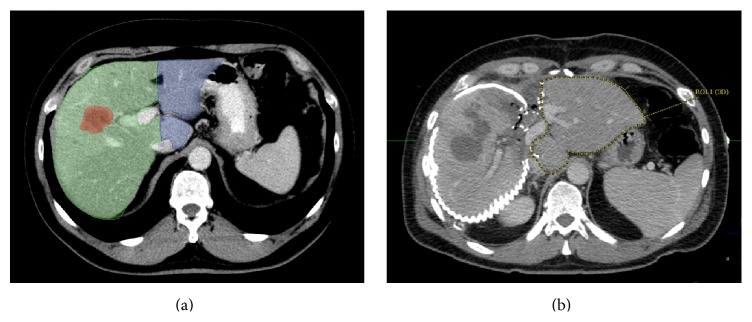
Pre- and postoperative CT-scan of the liver. Preoperative CT-scan depicting the tumor lesion (marked red) within the right liver lobe (a). Furthermore, the resected liver portion (marked green) and the resulting future liver remnant (FLR; marked blue) are shown. (b) CT-volumetry 10 days after the first step of ALPPS resulted in a significant increase of the FLR (marked by dotted yellow line). The extended right liver lobe (wrapped in a silicone matting) meanwhile showed signs of necrosis following ligation of the right portal vein.
